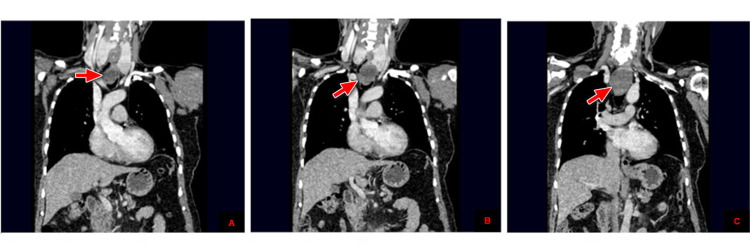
CT scan of the chest with contrast in the coronal plane with red arrows pointing to the thyroid mass in A and B. It extends from T1 to the T3 level, and no calcification or necrosis is seen within it (C).
